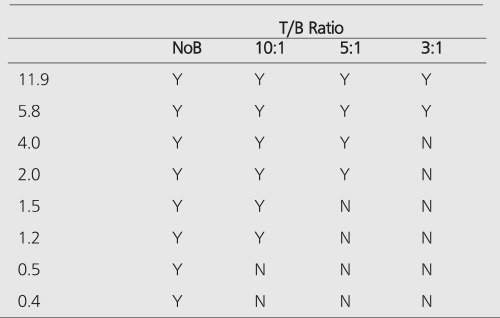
Limits of detection for planar Tc-99m imaging. Based on thesimilar findings in detection for I-131 SPECT and planar modalities,SPECT measurements for Tc-99m were not performed
